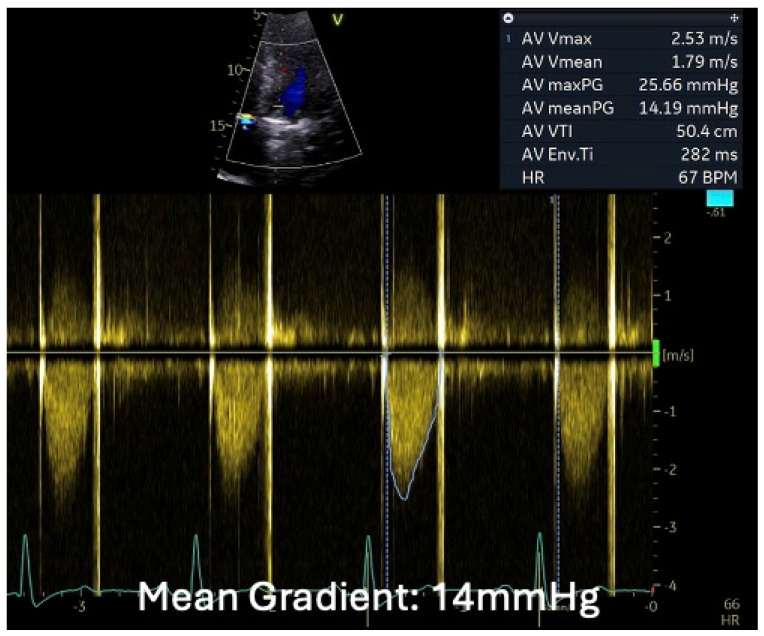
Transthoracic echocardiography—CW measurements of the transvalvular gradient across the mechanical AVR. After redoing the mechanical AVR and ascending Aorta replacement, the flow velocity across the mechanical AVR was normal.
